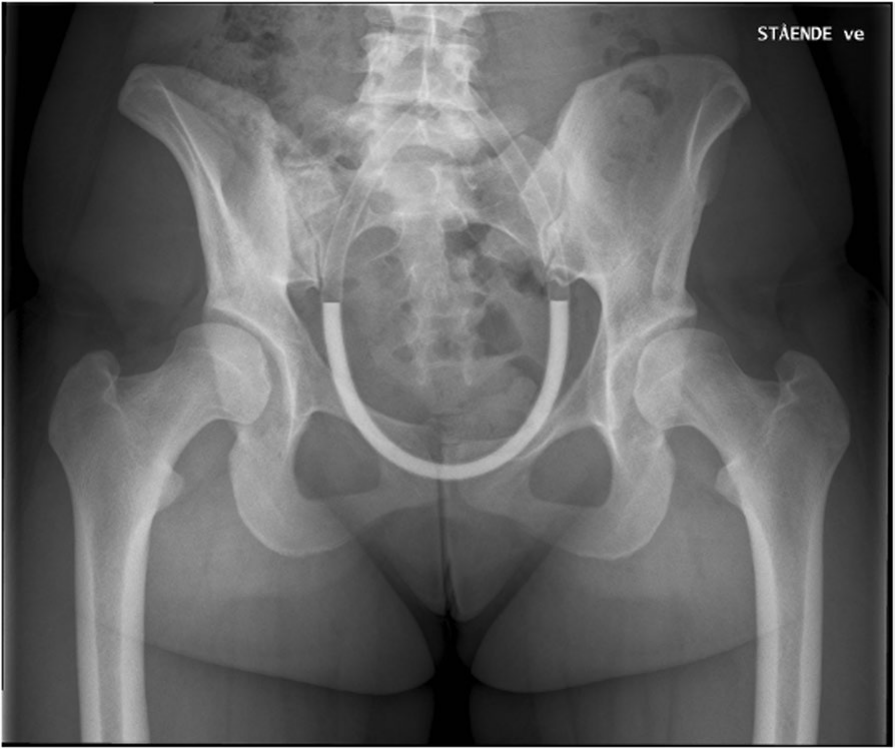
A weight-bearing anteroposterior radiograph of a study participant at skeletal maturity, revealing bilateral moderate dysplasia. The film/focus distance was 1.2 m and centered at 2 cm proximal to the symphysis, and hips were kept in a neutral abduction–adduction position with toes pointing forwards
